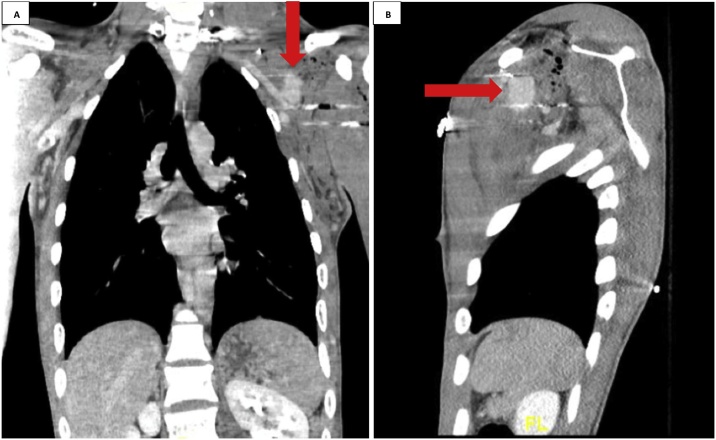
CT Angiography Chest. A. Coronal view: visualized above is a left subclavian/axillary artery injury with contrast extravasation at the site of injury along the course of the artery coursing out of the neck and in to the left shoulder. B. Sagittal view: a large amount of extravasation is seen in the left shoulder area along with an expanding hematoma. Subcutaneous emphysema is also visualized.
